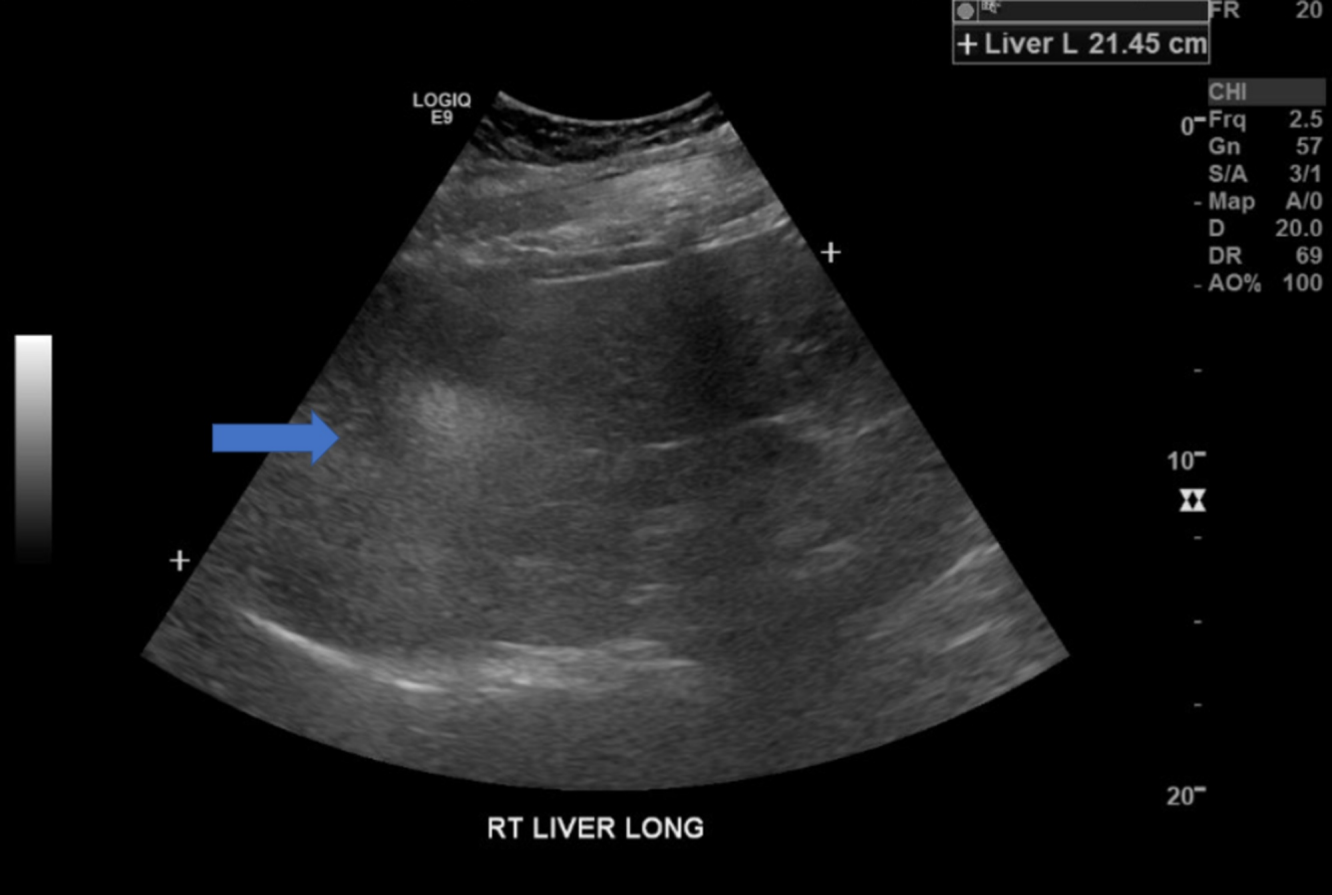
US of liver - Hepatic enlargement with associated fatty infiltration changes. US: ultrasound
